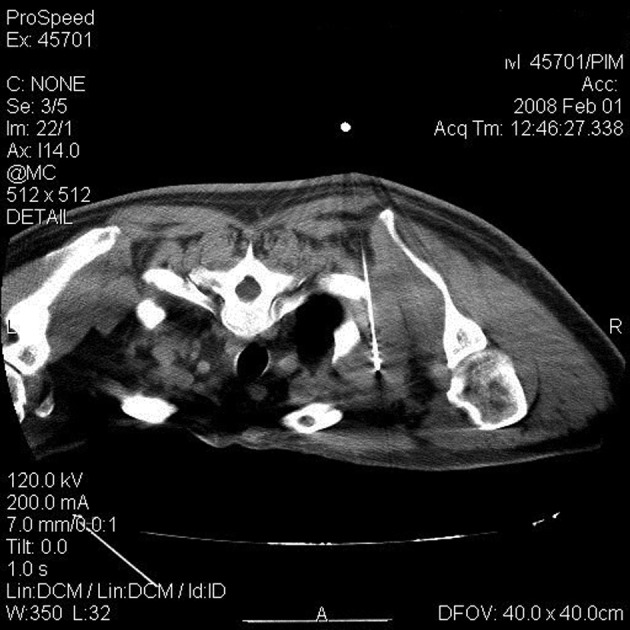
Computed tomography (CT)-guided radiofrequency ablation (RFA) probe in the right supraclavicular soft-tissue mass (diameter, 6×7 cm). The scan was taken with the patient in the prone position.
